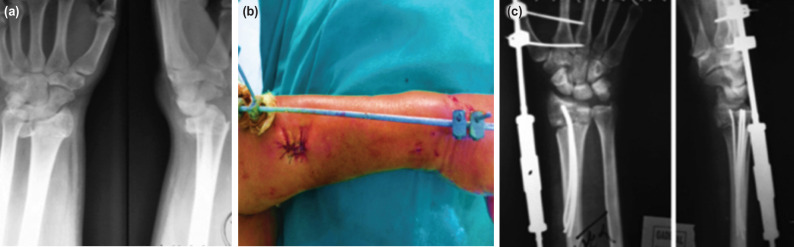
(a) AP and lateral radiographic images showing of a case with osteoporosis and comminuted distal radial fracture, (b) clinical photograph showing placement of an external fixator, (c) Radiographic views following final placement of IM wires and external fixator.
